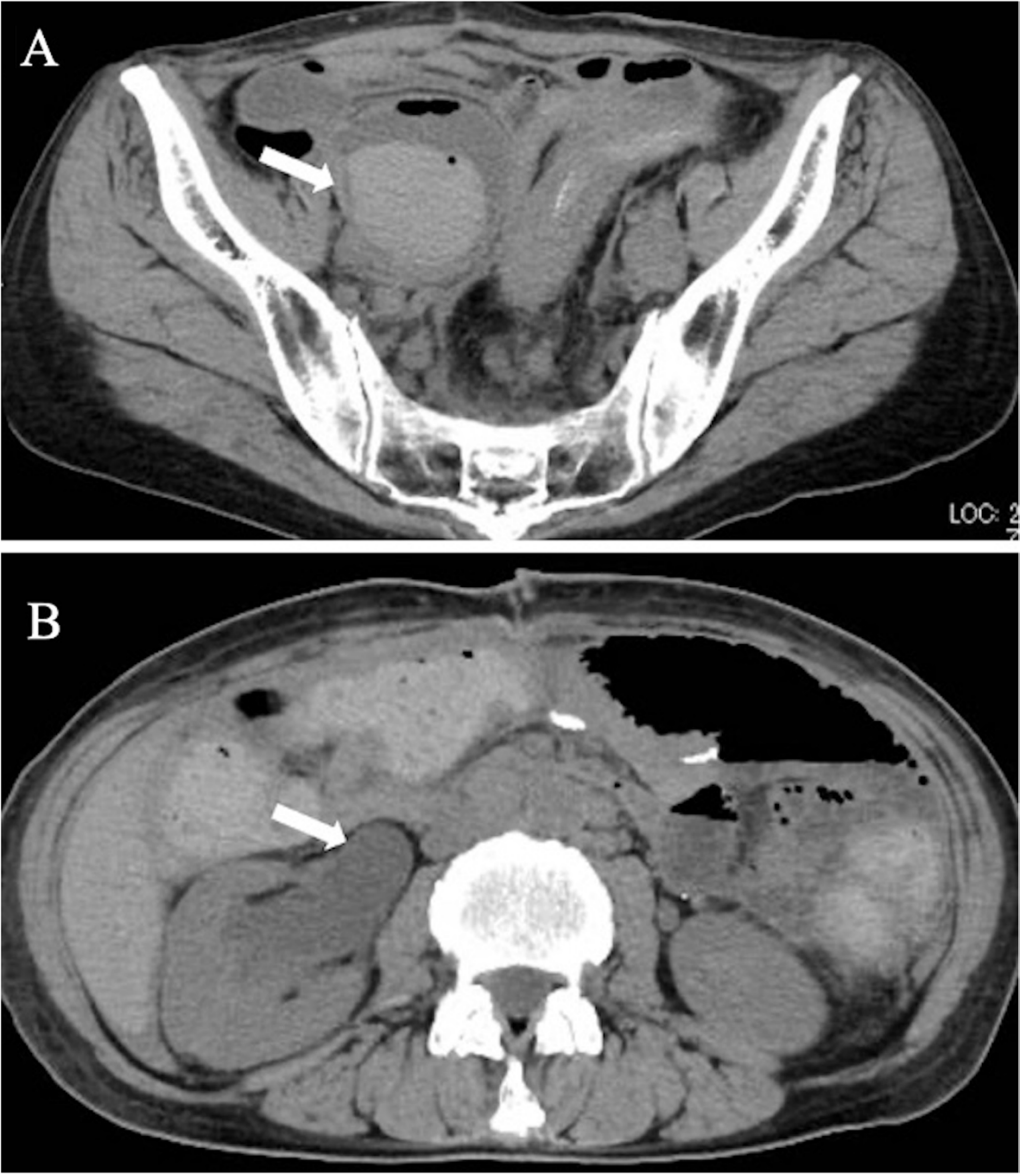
Noncontrast-enhanced computed tomography shows (A) a massive hematoma in the right ureter and bladder (arrow) and (B) right hydronephrosis (arrow)
